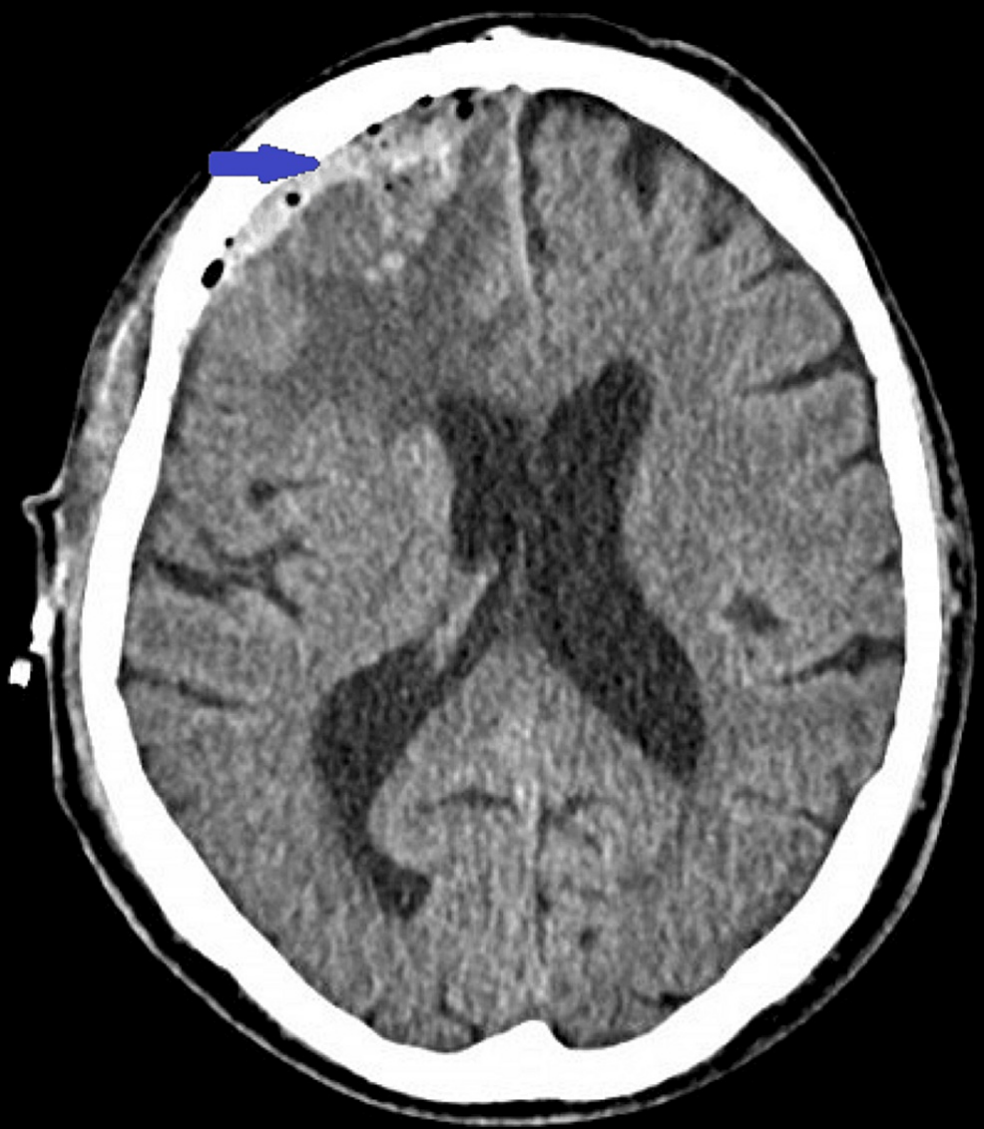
Postoperative brain CT showing a thin pellicle of subdural hemorrhagic collection (blue arrow) in the background of persistent edema of the frontal lobe of the right cerebral hemisphere.
